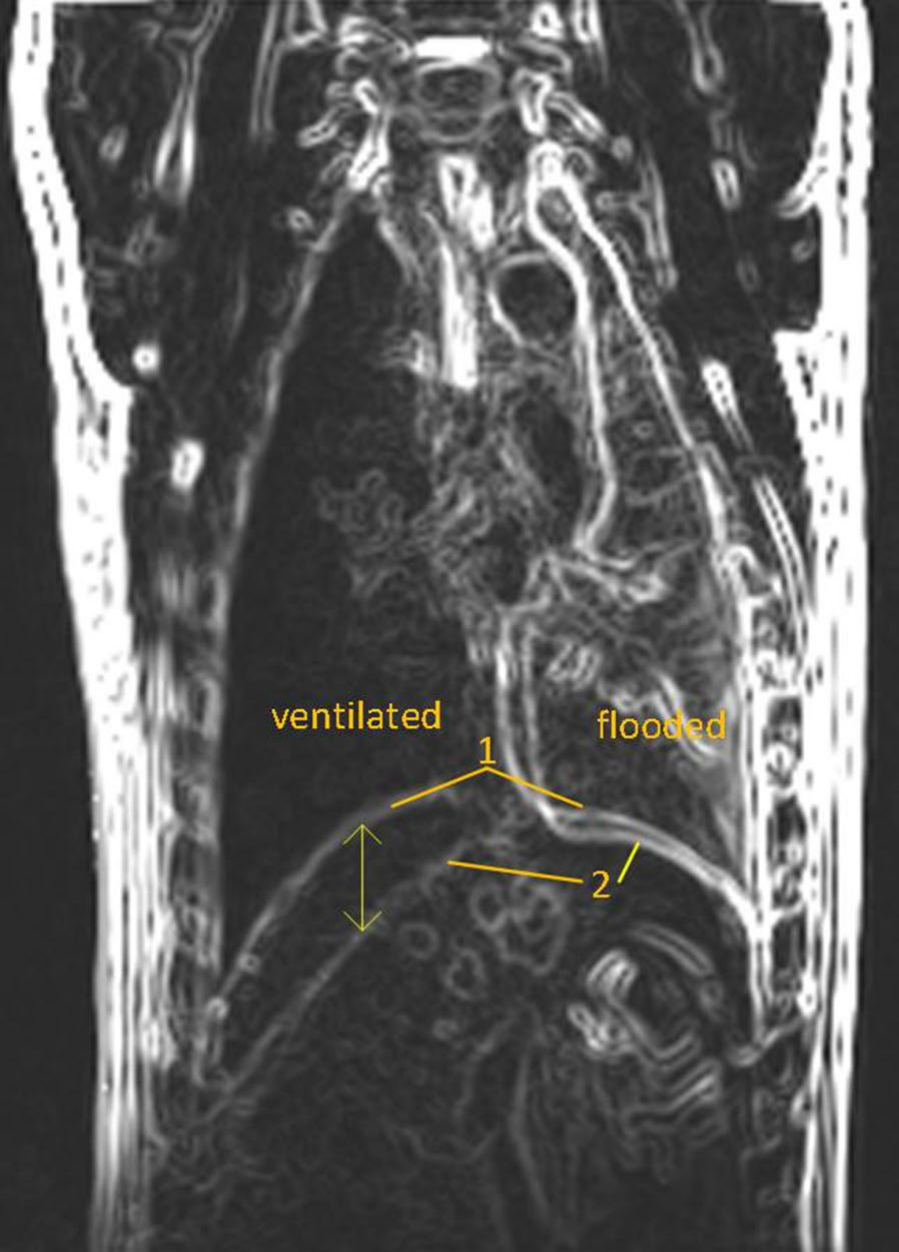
MR image merged from end-expiration and end-inspiration after edge detection; localisation of the flooded lung left and ventilated lung right with diaphragm position in end-expiration (*1*) and end-inspiration (*2*). *Arrow* indicates diaphragm displacement during breath cycle in the frontal plane
